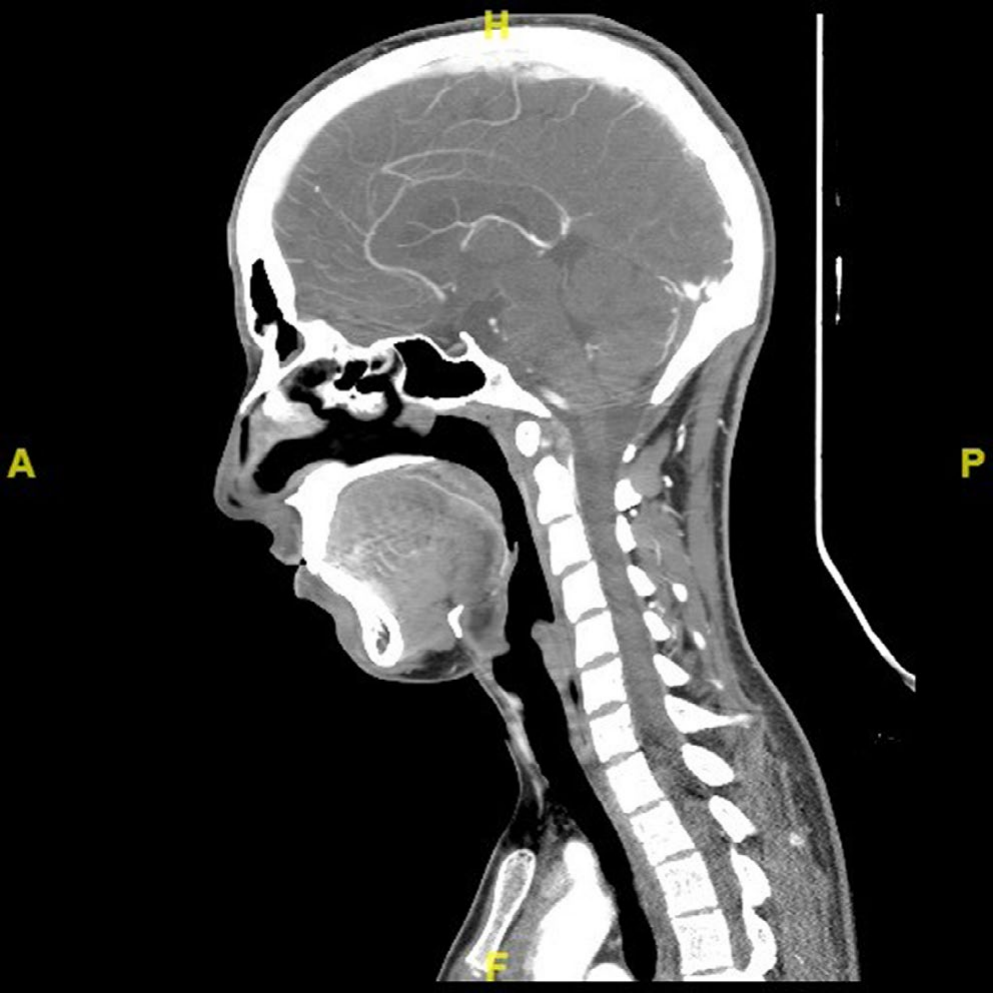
CT angiogram of the neck vessels and intracranial circulation with no significant findings
